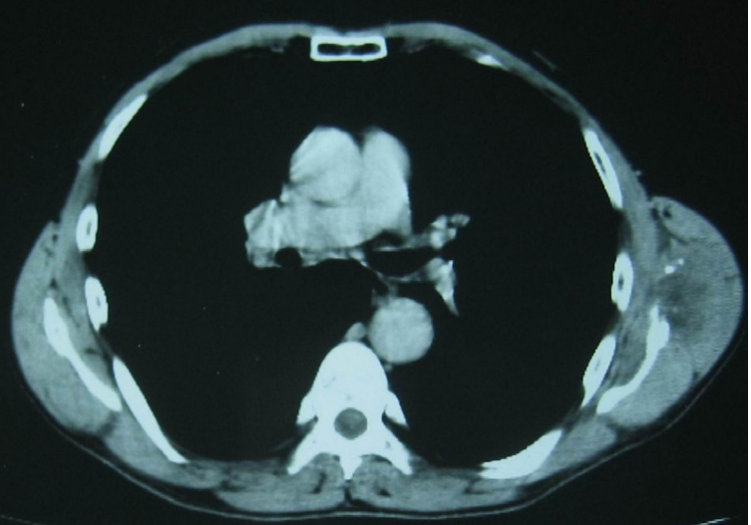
Axial CT scan revealed a soft tissue mass localized in the lower scapular region with intralesional calcifications and a secondary osseous invasion of the lower scapula.
